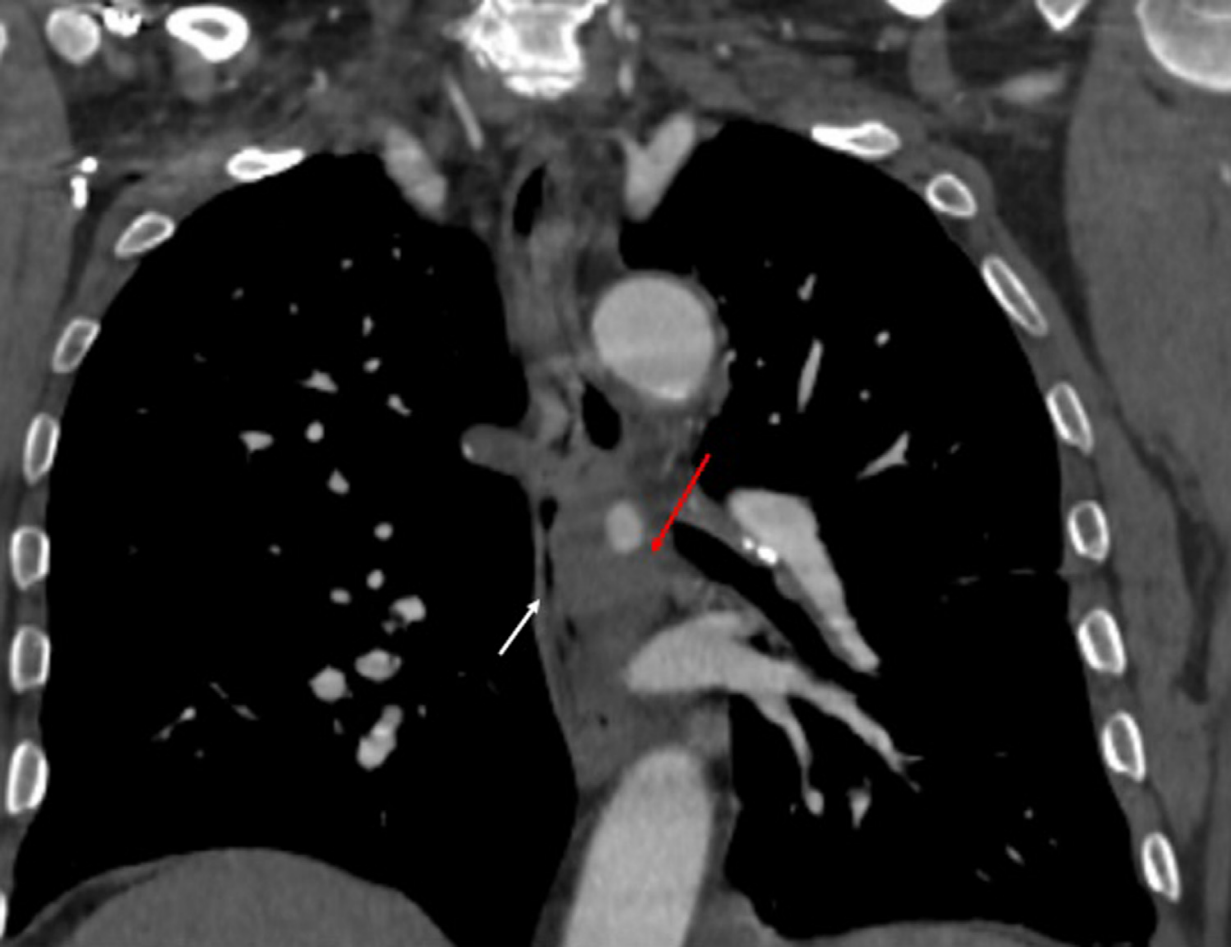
Coronal CT scan view showing the relation of the aneurysm with mediastinal structures. It is displacing the esophagus (red arrow). to the right.
